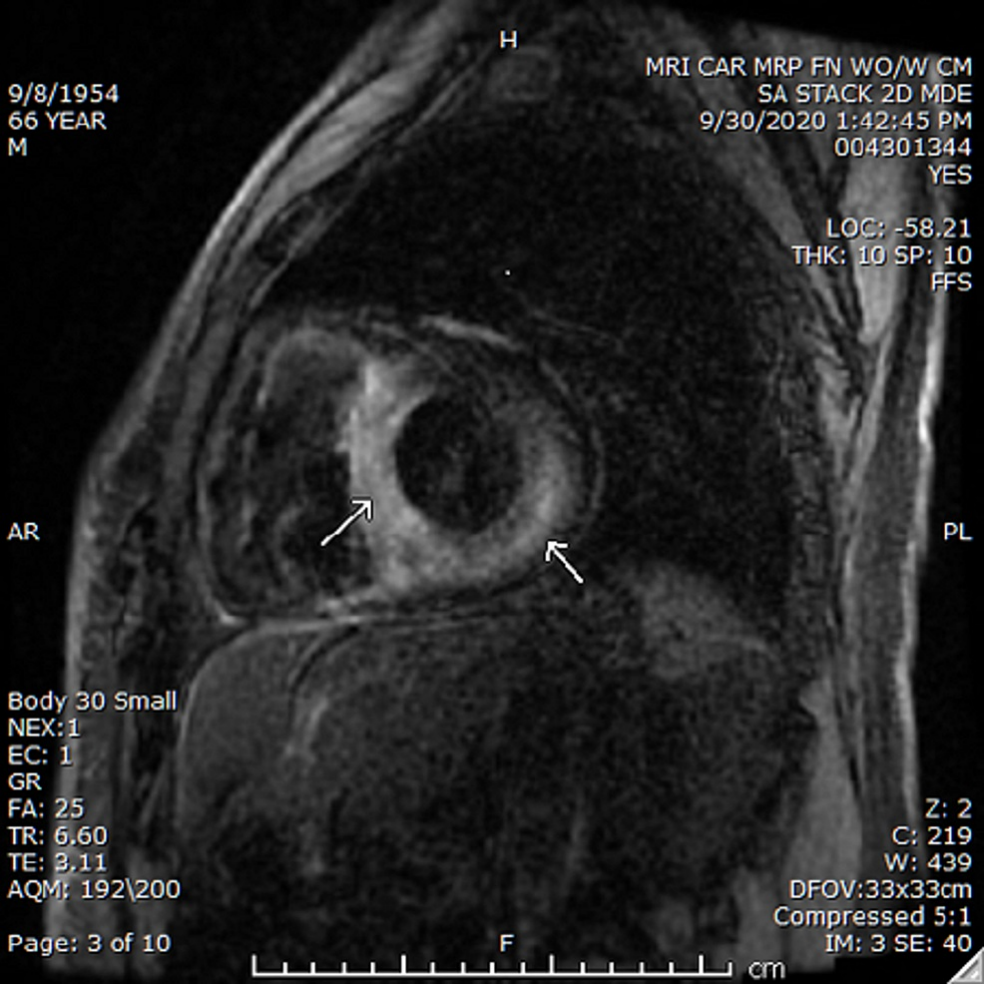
MRI of the heart with delayed gadolinium enhancement showing an enlarged heart with transmural delayed enhancement of the septal and lateral walls of the left ventricle; additional patchy subendocardial and mid-myocardial delayed enhancement is also seen involving the anterolateral wall.
